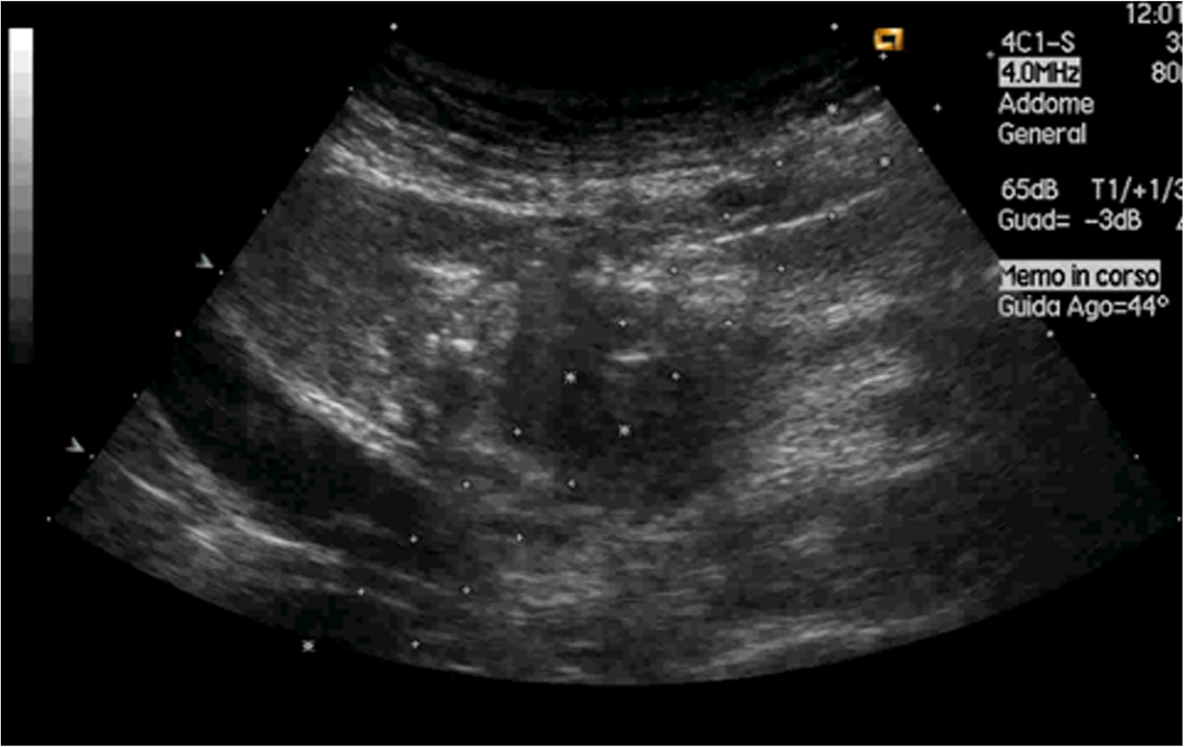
Ultrasound-guided pancreatic lesion biopsy. The path of the needle can be precisely visualised during the planning phase (*dotted line*). The tip of the needle can be exactly visualised during its insertion and stopped when in the target lesion (*hyperechoic spot*)
